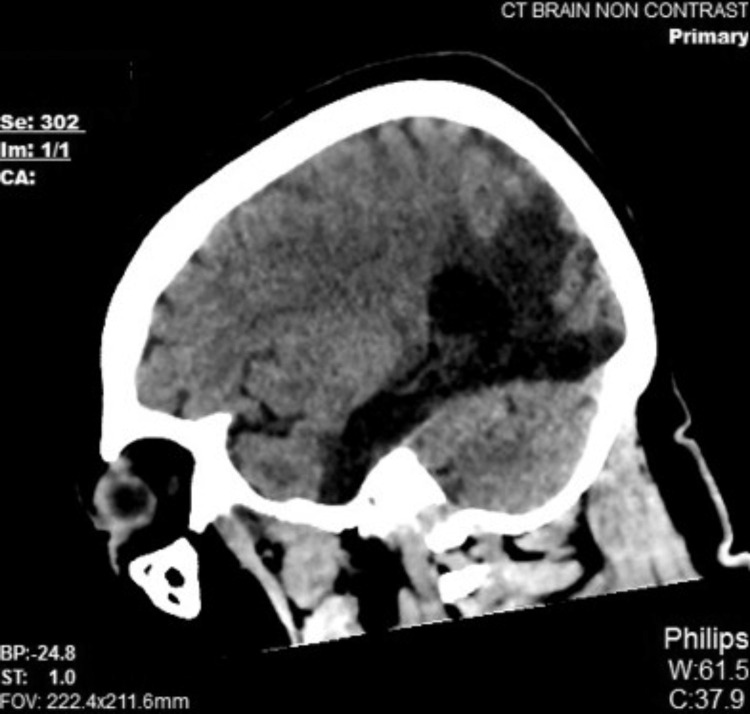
CT of the brain in the sagittal plane (multiplanar reconstruction) demonstrated the extent of the encephalomalacic changes. CT: computed tomography.
